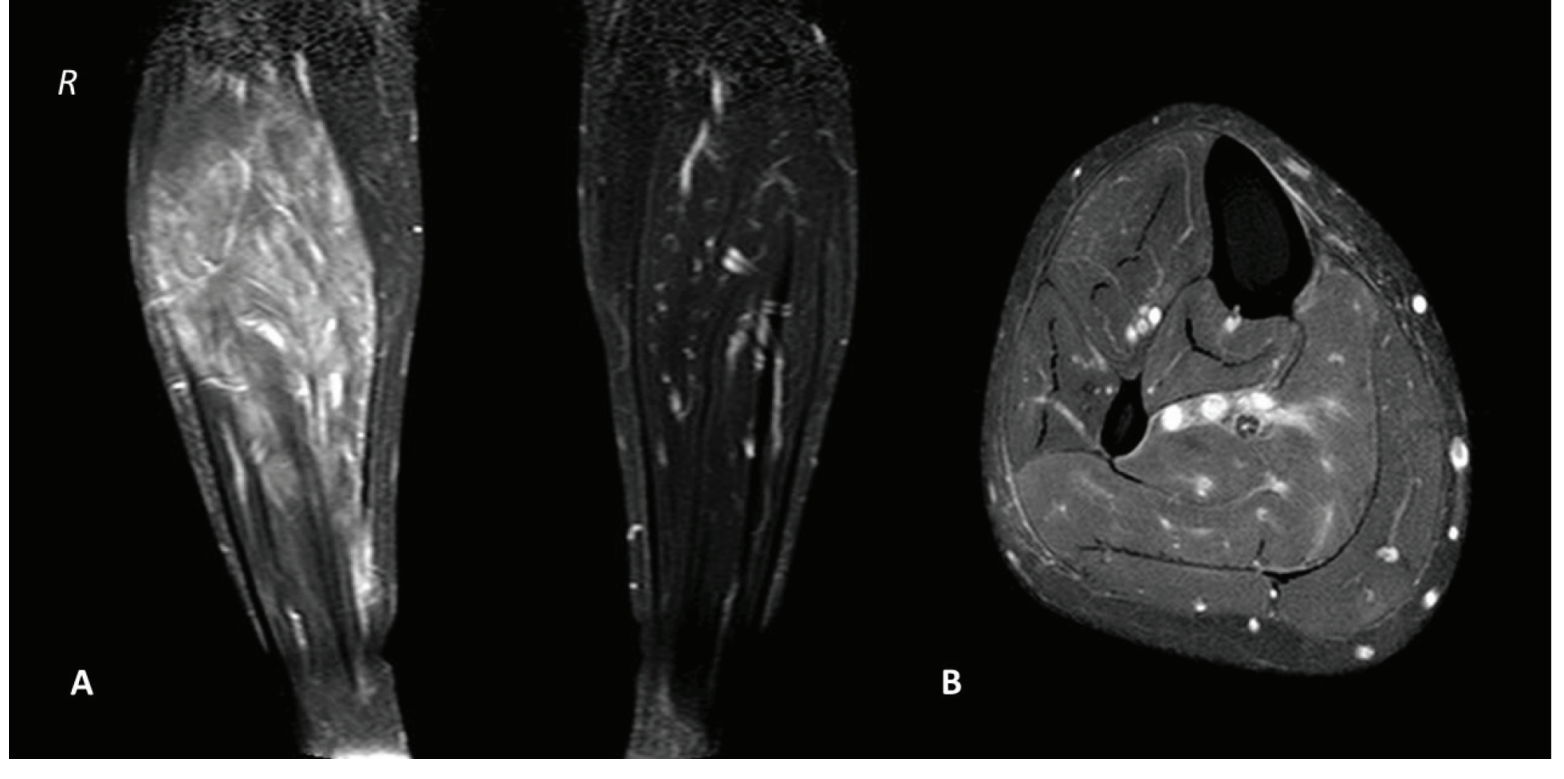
(A) Coronal and (B) axial T2-weighted fat saturation magnetic resonance images of the right calf muscles showing increased intensity and severe swelling due to muscular inflammation.
